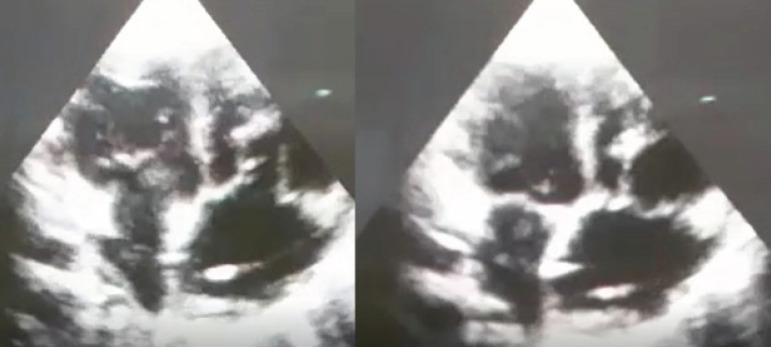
Postsurgical echocardiogram of the replacement of the tricuspid valve with an autologous pericardium.
